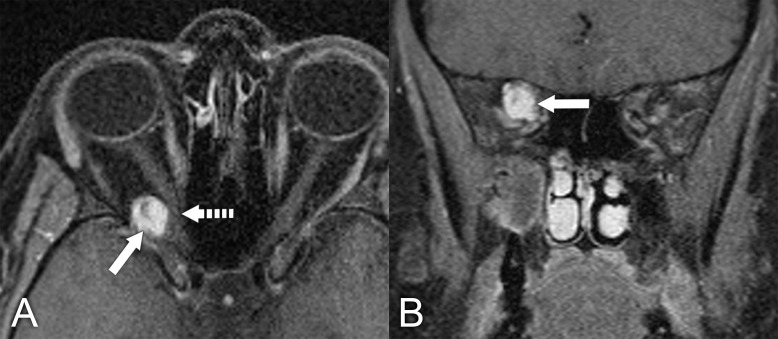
Magnetic resonance imaging findings in a 47-year-old woman who presented with a gradual onset of blurry vision in the right eye. (A) Axial T1 postcontrast MRI with fat saturation shows a heterogeneously enhancing mass in the right orbital apex measuring 1.3 cm × 1.1 cm × 0.9 cm (solid arrow) with compression of the optic nerve (dashed arrow). (B) Delayed coronal T1 postcontrast MRI with fat saturation shows gradual filling of contrast in the lesion (arrow). The presumed diagnosis of cavernous venous malformation was made based on the characteristic radiologic findings. The patient was treated with stereotactic radiotherapy, and her visual function improved in response to this treatment.
